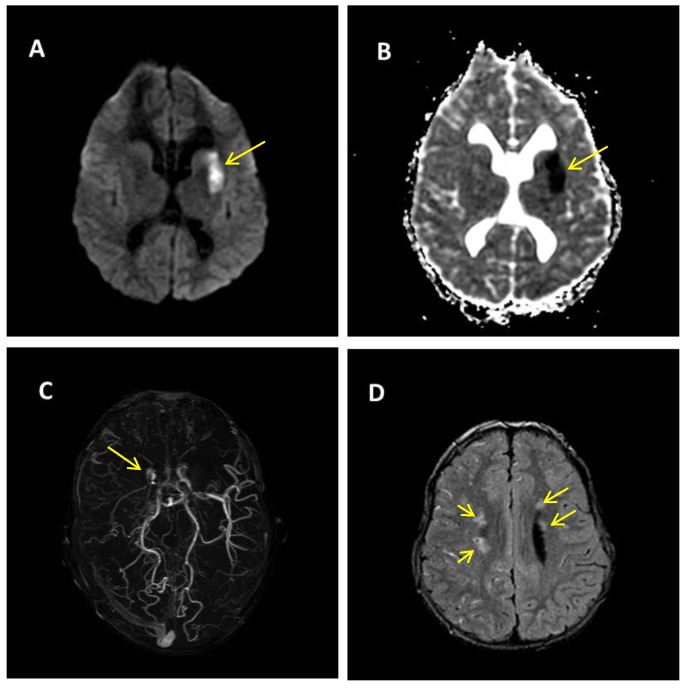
Brain magnetic resonance imaging (MRI) at the age of 10 years, after the first TI sequence. (A,B) Diffusion weighted imaging (A) and diffusion coefficient map (B) show a lesion of the left lenticular nucleus of the anterior part of the internal capsula, and the head and body of the lateral nucleus caudatus characterised by reduced diffusion due to an acute ischemic lesion (yellow arrows). (C) Angio-TOF MRI showing the stenosis of the right carotid siphon apex (yellow arrow) associated with poorly visualised flow of the ipsilateral middle cerebral artery, stenosis of the left carotid siphon apex, and a stretch of the M1 ipsilateral middle cerebral artery; (D) Fluid attenuation inversion recovery (FLAIR) sequence: small areas of signal hyperintensity at the semioval centres bilaterally (yellow arrows), most marked on the right side.
